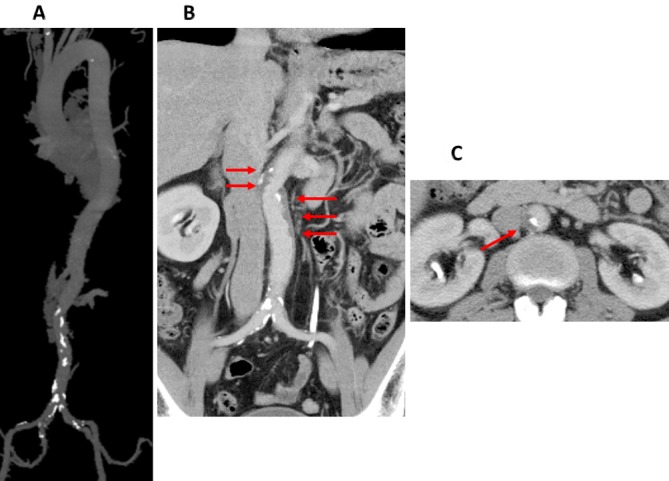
CT angiography images of the aorta. (A) Maximum intensity projection. Calcification deposited in the infrarenal abdominal aorta; however, a few calcifications were found in other sections. (B) Coronal view of the abdominal aorta. Red arrows show the false lumen. (C) Axial image at the T12–L1 level. Red arrows show the false lumen with calcification.
